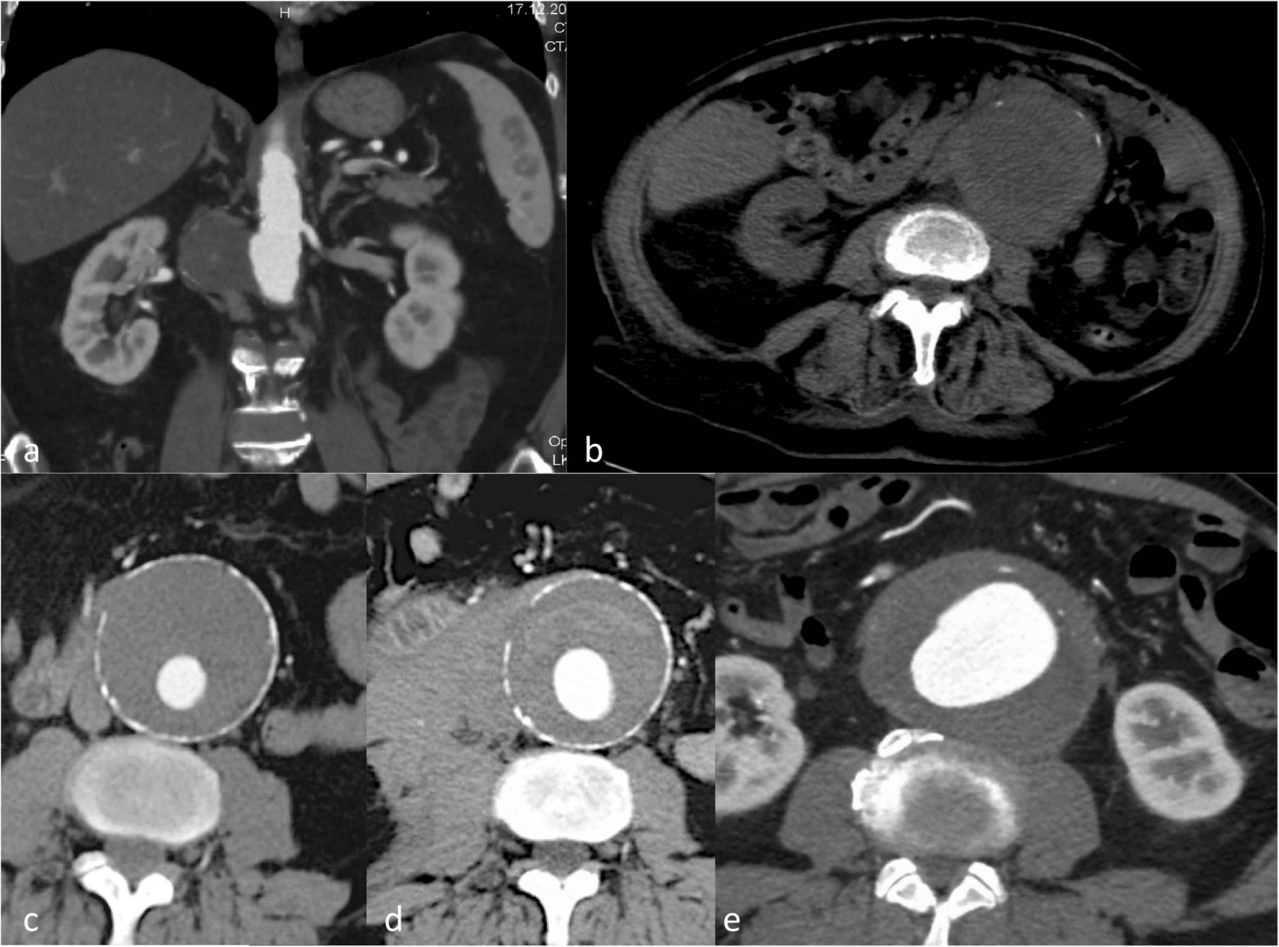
Signs of impending rupture. Focal “blebbing” (*arrow*) of the aortic wall (a, c). “Crescent sign” with hyperdense thrombus (b). Draped aorta signs (b, e) with flattening of the posterior aspect of the abdominal aorta. Focal “discontinuation” of the aortic contour (here with discontinuation of the wall calcification and blebbing) (c) in a 6-cm abdominal aortic aneurysm. The patient refused further treatment. He presented with hypotension 3 months later. CTA demonstrated a ruptured aneurysm (d)
